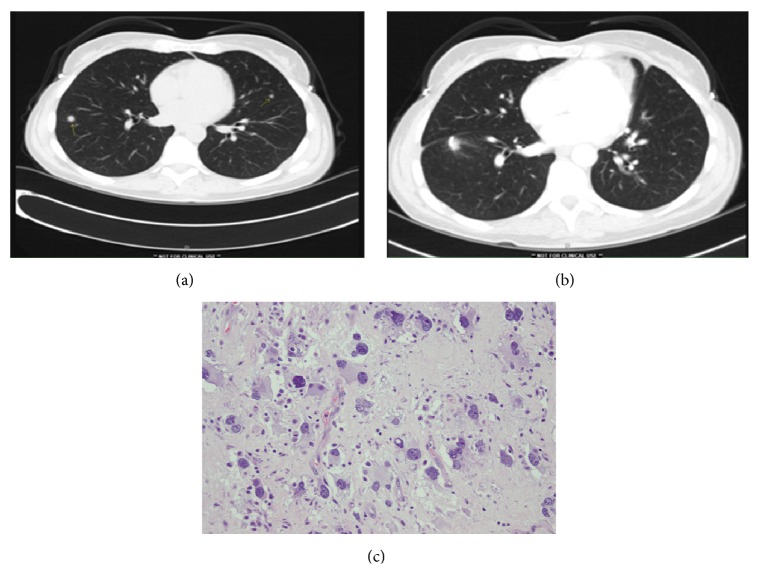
Pre- and postsurgery CAT scan of a 46-year-old lady with history of dedifferentiated liposarcoma patient who completed the DC vaccine + RT treatment, underwent surgical resection, and approximately 10 months later presented with (a and b) pre- and post-CT scans showing the presence of the lung nodules (two) and then postsurgical imaging study after its resection. (c) Pathological analysis showed tissue necrosis, hyalinization, and infiltration of lymphocytes and is currently free of systemic or local recurrence over 8 years.
